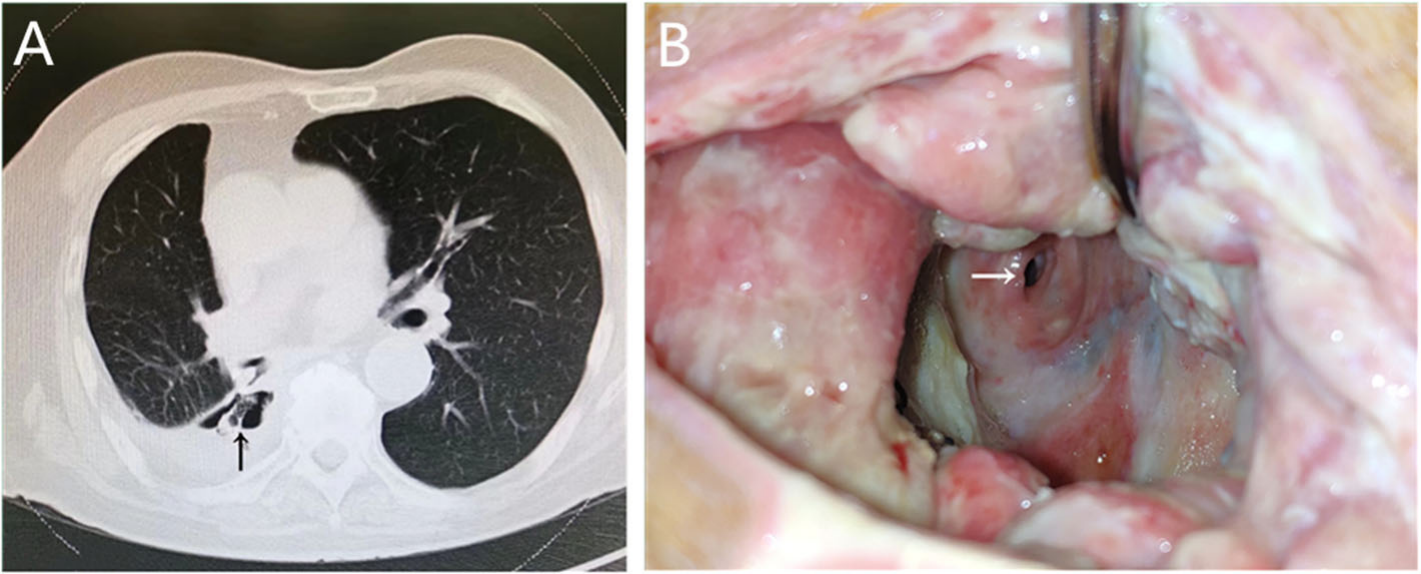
A Chest CT shows that the bronchial stent was displaced and dropped into the abscess cavity (black arrow). B After changing the dressings for 4 weeks, the cavity wall was clean, and the granulation tissue was fresh. A BPF with a diameter of approximately 8 mm can be seen (white arrow)
